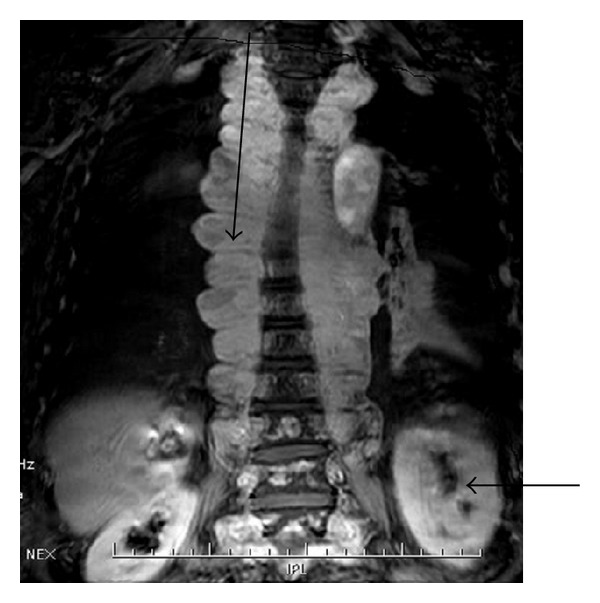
MRI shows asymmetrical soft tissue mass with a stringy appearance surrounding the thoracic vertebral bodies. On November 15, 2009, an MRI was performed. The upper arrow indicated soft tissue masses with a stringy appearance surrounding the thoracic vertebral bodies, and the lower arrow suggested a soft tissue mass in the left kidney.
